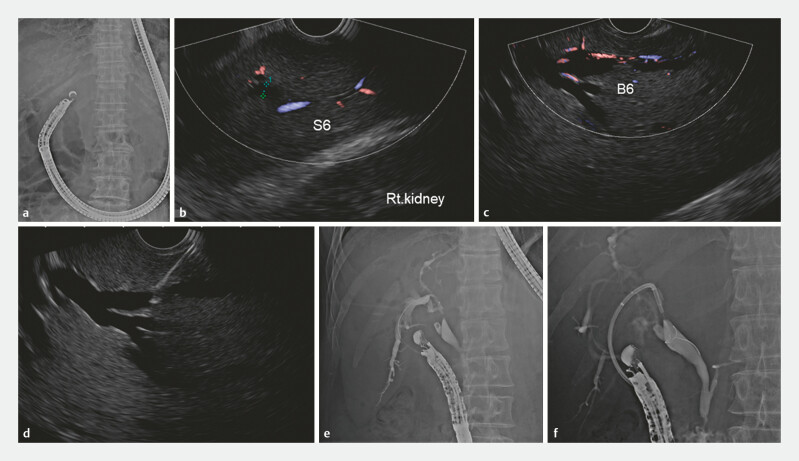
Method of endoscopic ultrasound-guided hepaticoduodenostomy (EUS-HDS).
